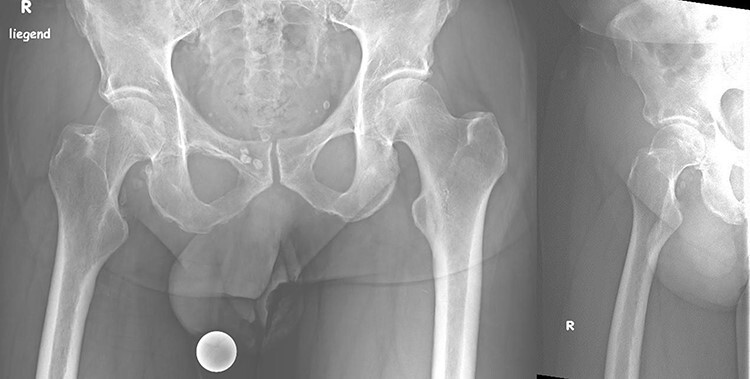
One-year postoperative radiograph: pelvic overview and Lauenstein view.
